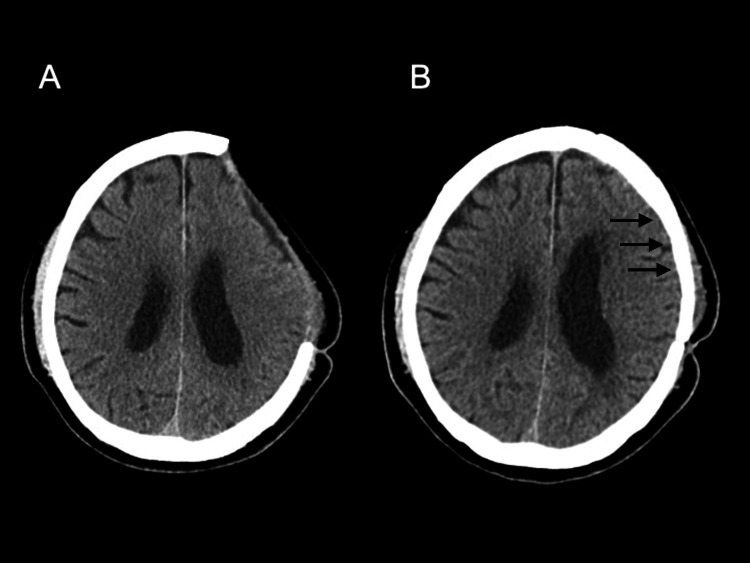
Perioperative head CT for cranioplasty. (A) Preoperative head CT obtained prior to cranioplasty. (B) No postoperative hemorrhagic complications observed following cranioplasty (solid arrows).
